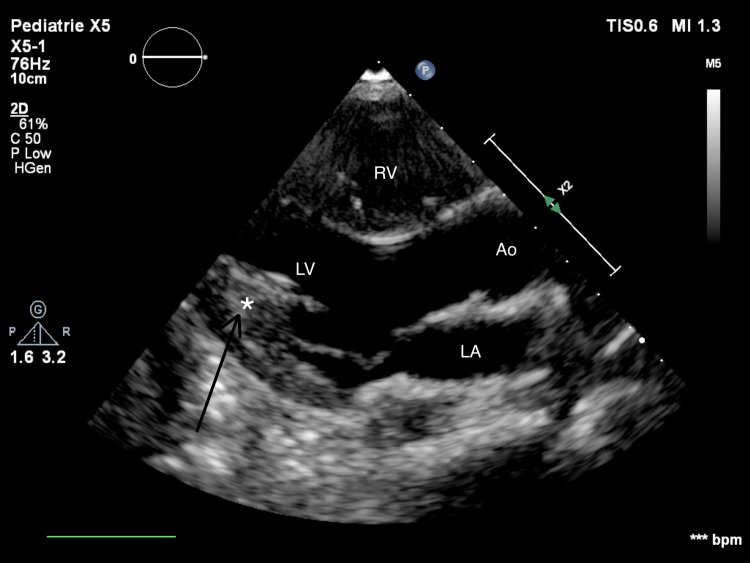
Cardiac ultrasound Hyperechogenic appearance of the myocardium (arrow)
